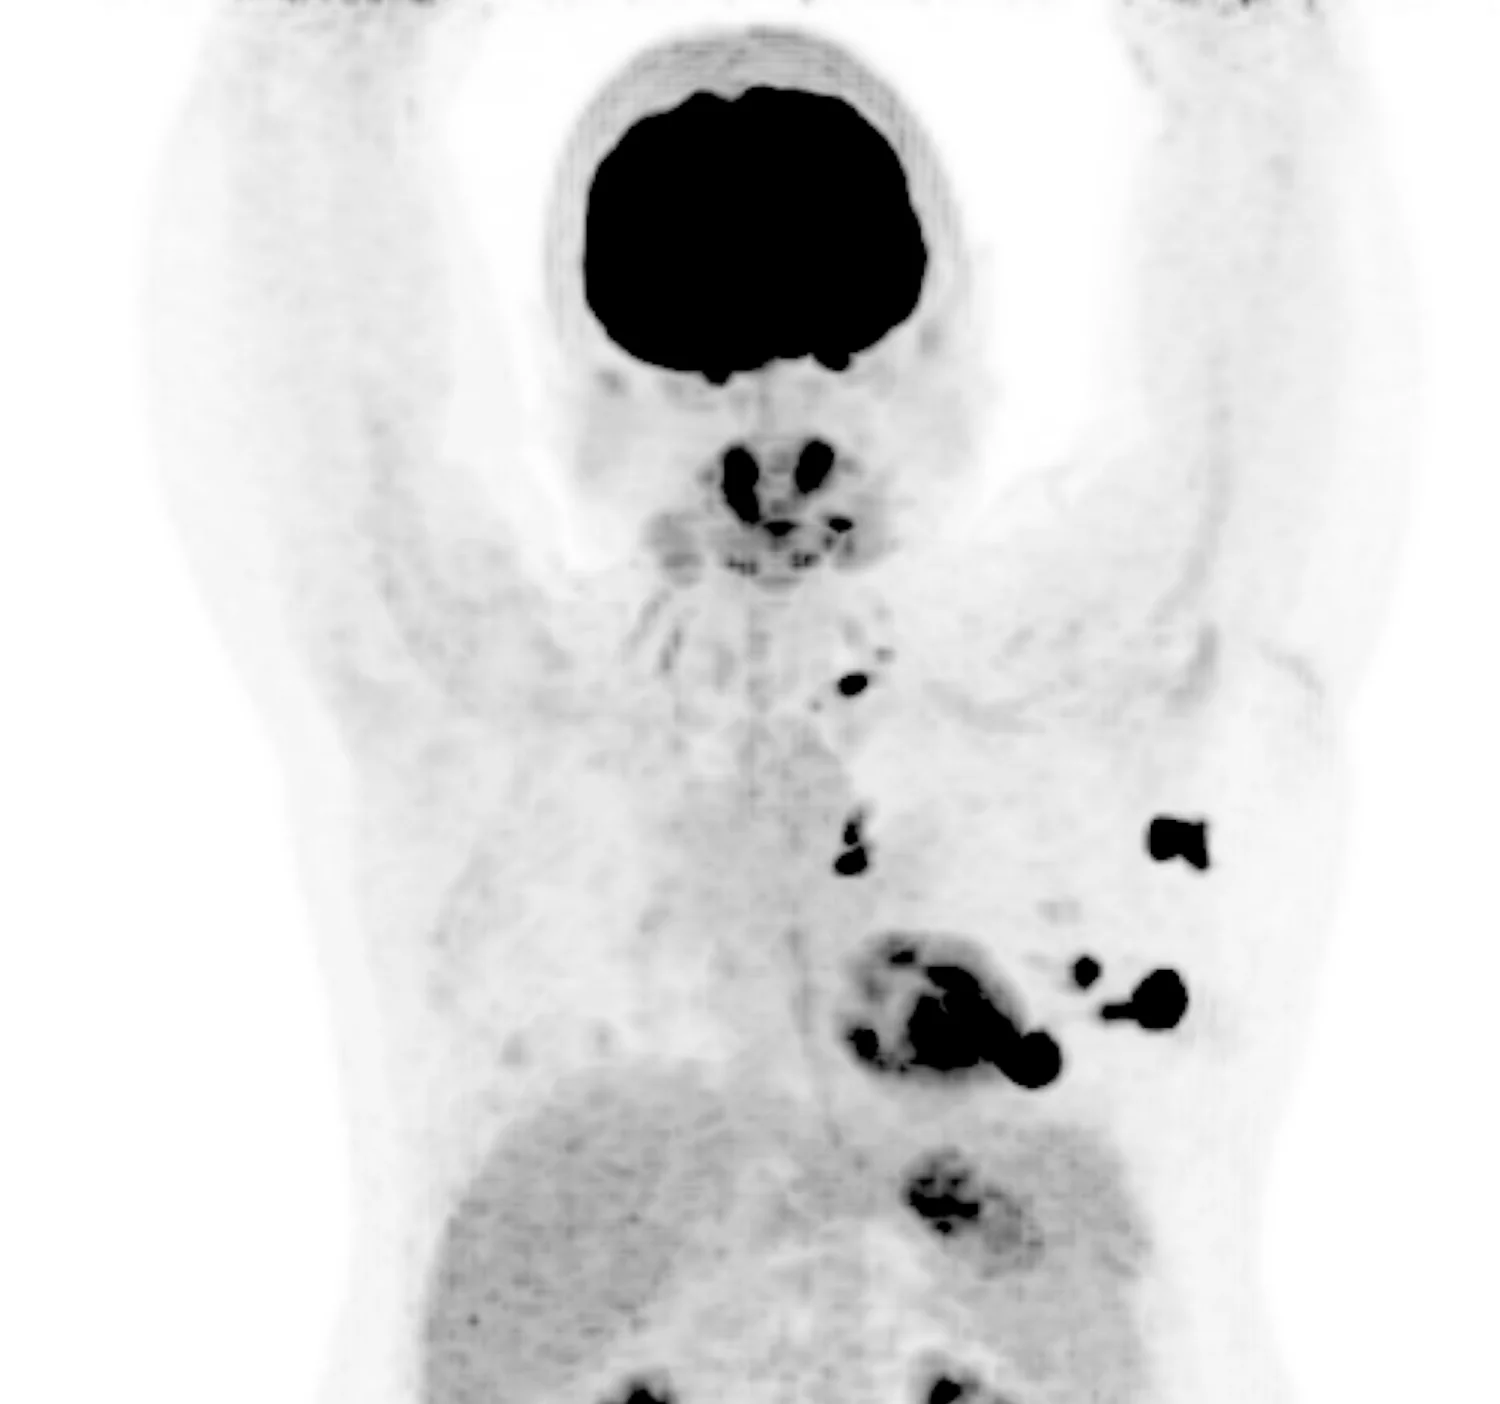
18 F-FDG PET/CT showing intense uptake in the primary tumor of the left breast and in left axillary level I–III, internal mammary, and supraclavicular lymph nodes
